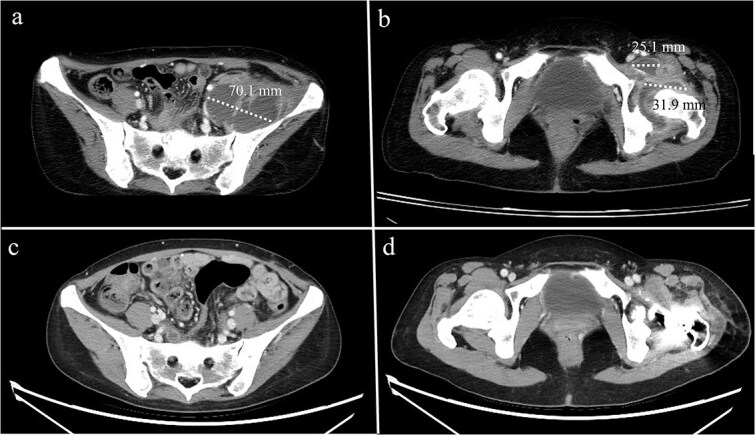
Computed tomography of a 44-year-old female demonstrating (a) a psoas abscess and (b) hip septic arthritis. Postdebridement surgery and resection arthroplasty, the abscess completely resolved (c, d).
